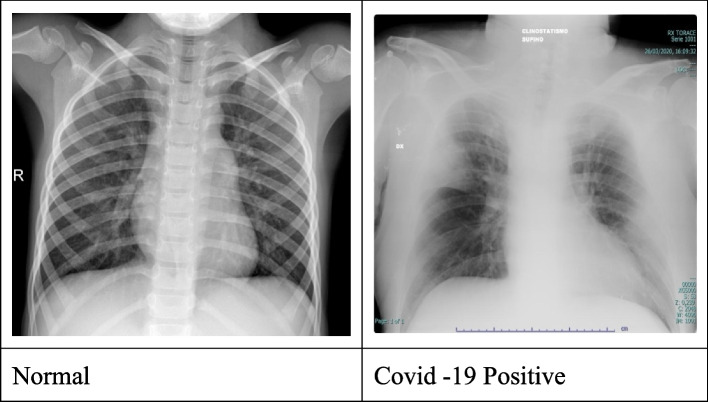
Dataset briefing of X-Rays under norma and abnormal representation
